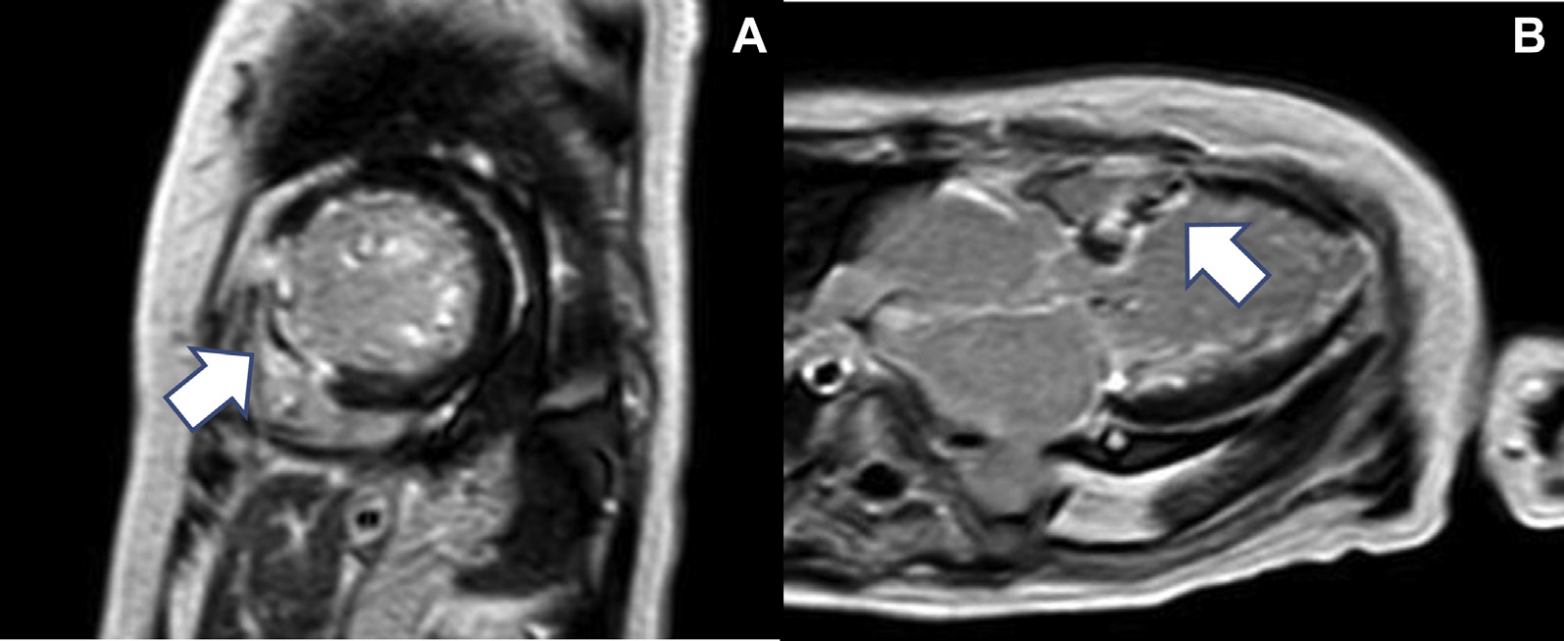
Cardiac Magnetic Resonance Imaging Findings After Percutaneous Transluminal Septal Myocardial Ablation Cardiac magnetic resonance imaging showing late gadolinium enhancement (white arrows) in the interventricular septum on (A) the short-axis view and (B) 3-chamber long-axis view.
